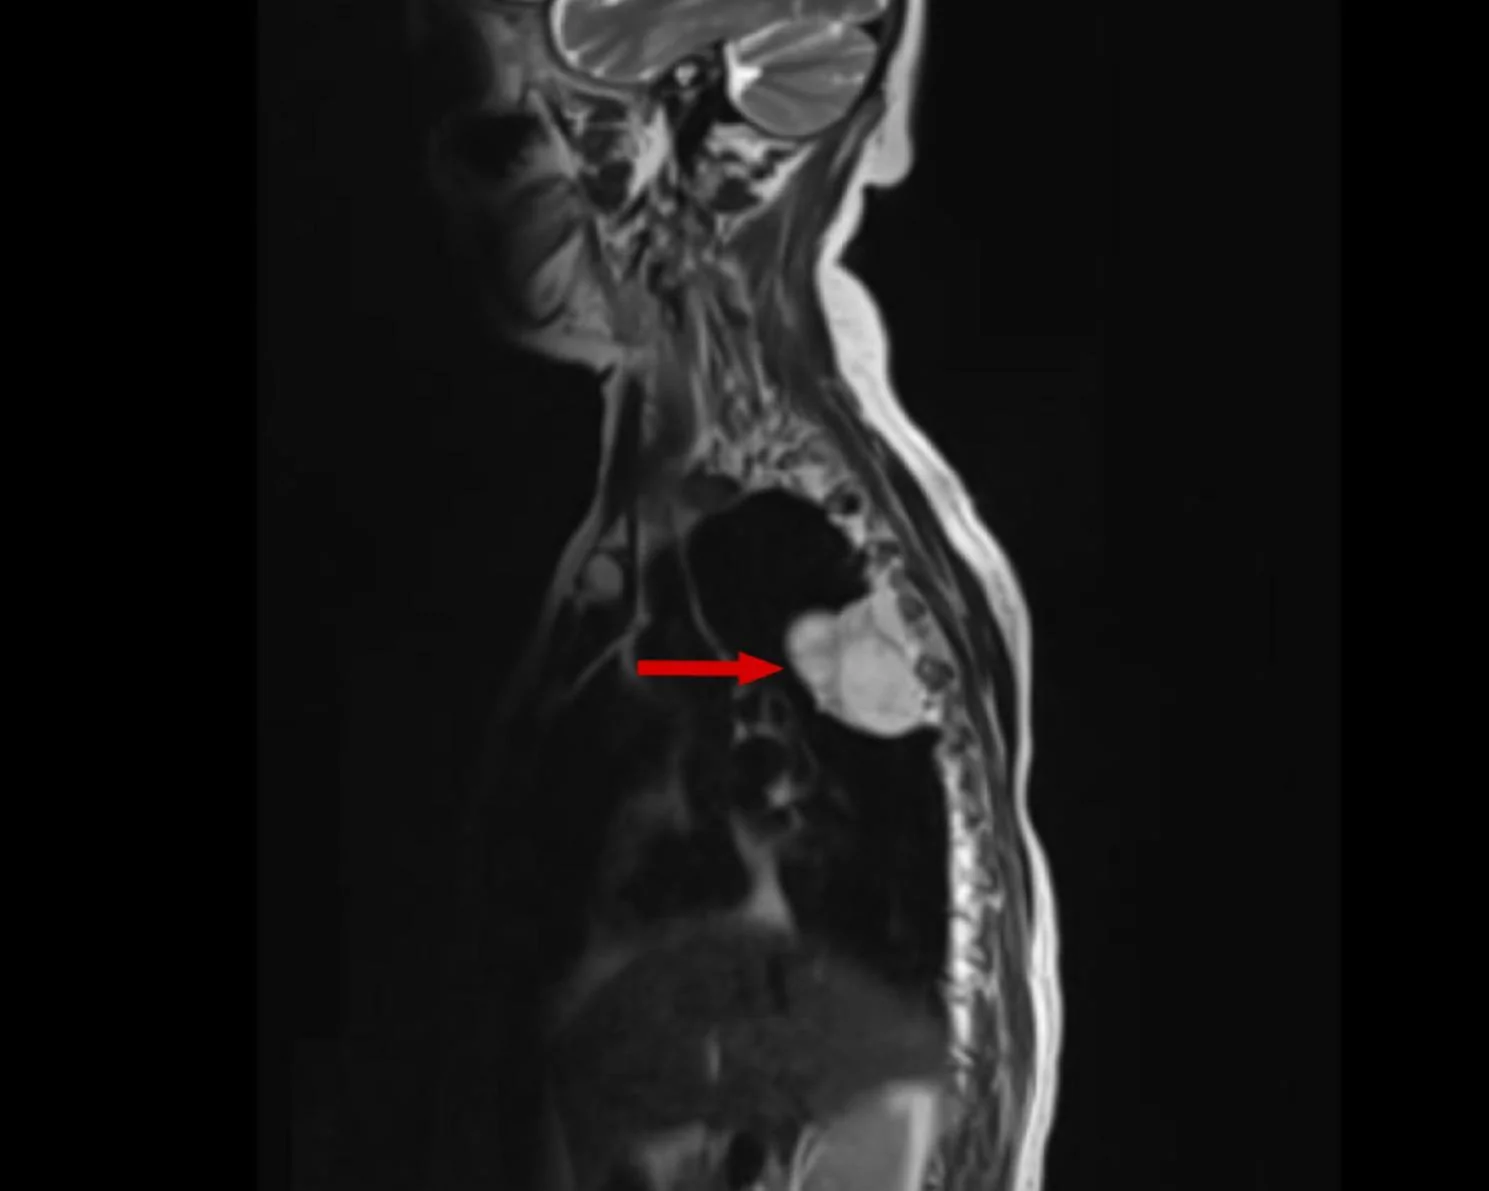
Preoperative imaging of the Throracic Dumbell Capillary Hemangioma. Sagittal T2-weighted MRI showing the hyperintense, lobulated, dumbbell-shaped epidural mass (arrow) causing severe compression of the spinal cord at the D3-D5 level
